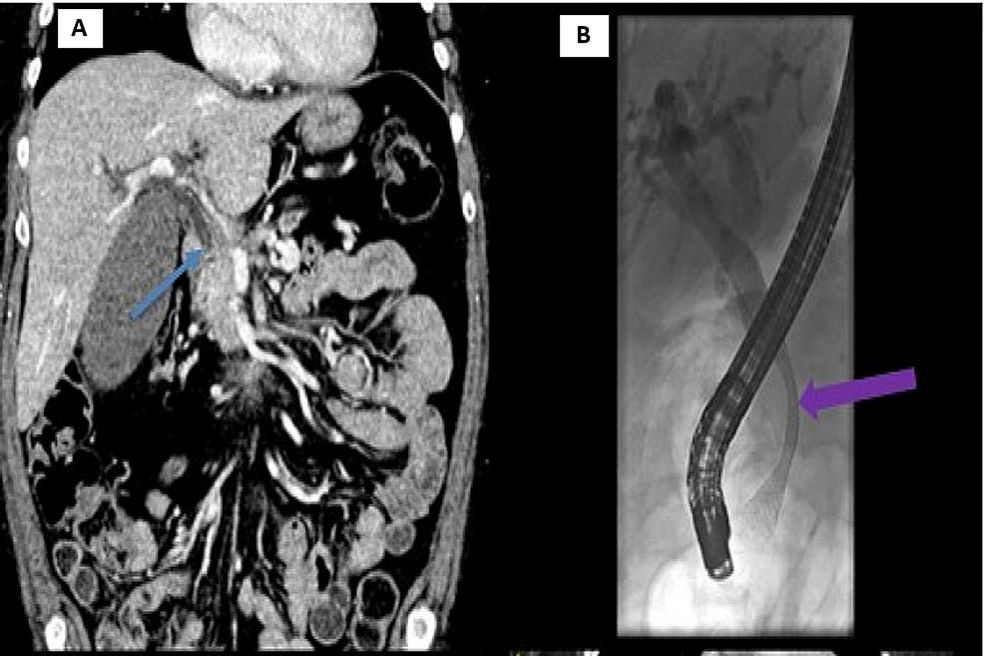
(A) Coronal CT of the abdomen with portal venous contrast, showing the initial presentation with dilated CBD and distinct transition (blue arrow), indicating obstruction secondary to a pancreatic head mass. (B) X-ray exposure from ERCP procedure. The patient was treated with an uncovered stent (purple arrow) deployed in the CBD via ERCP. CT, Computed tomography; CBD, common bile duct; ERCP, endoscopic retrograde cholangiopancreatography.
